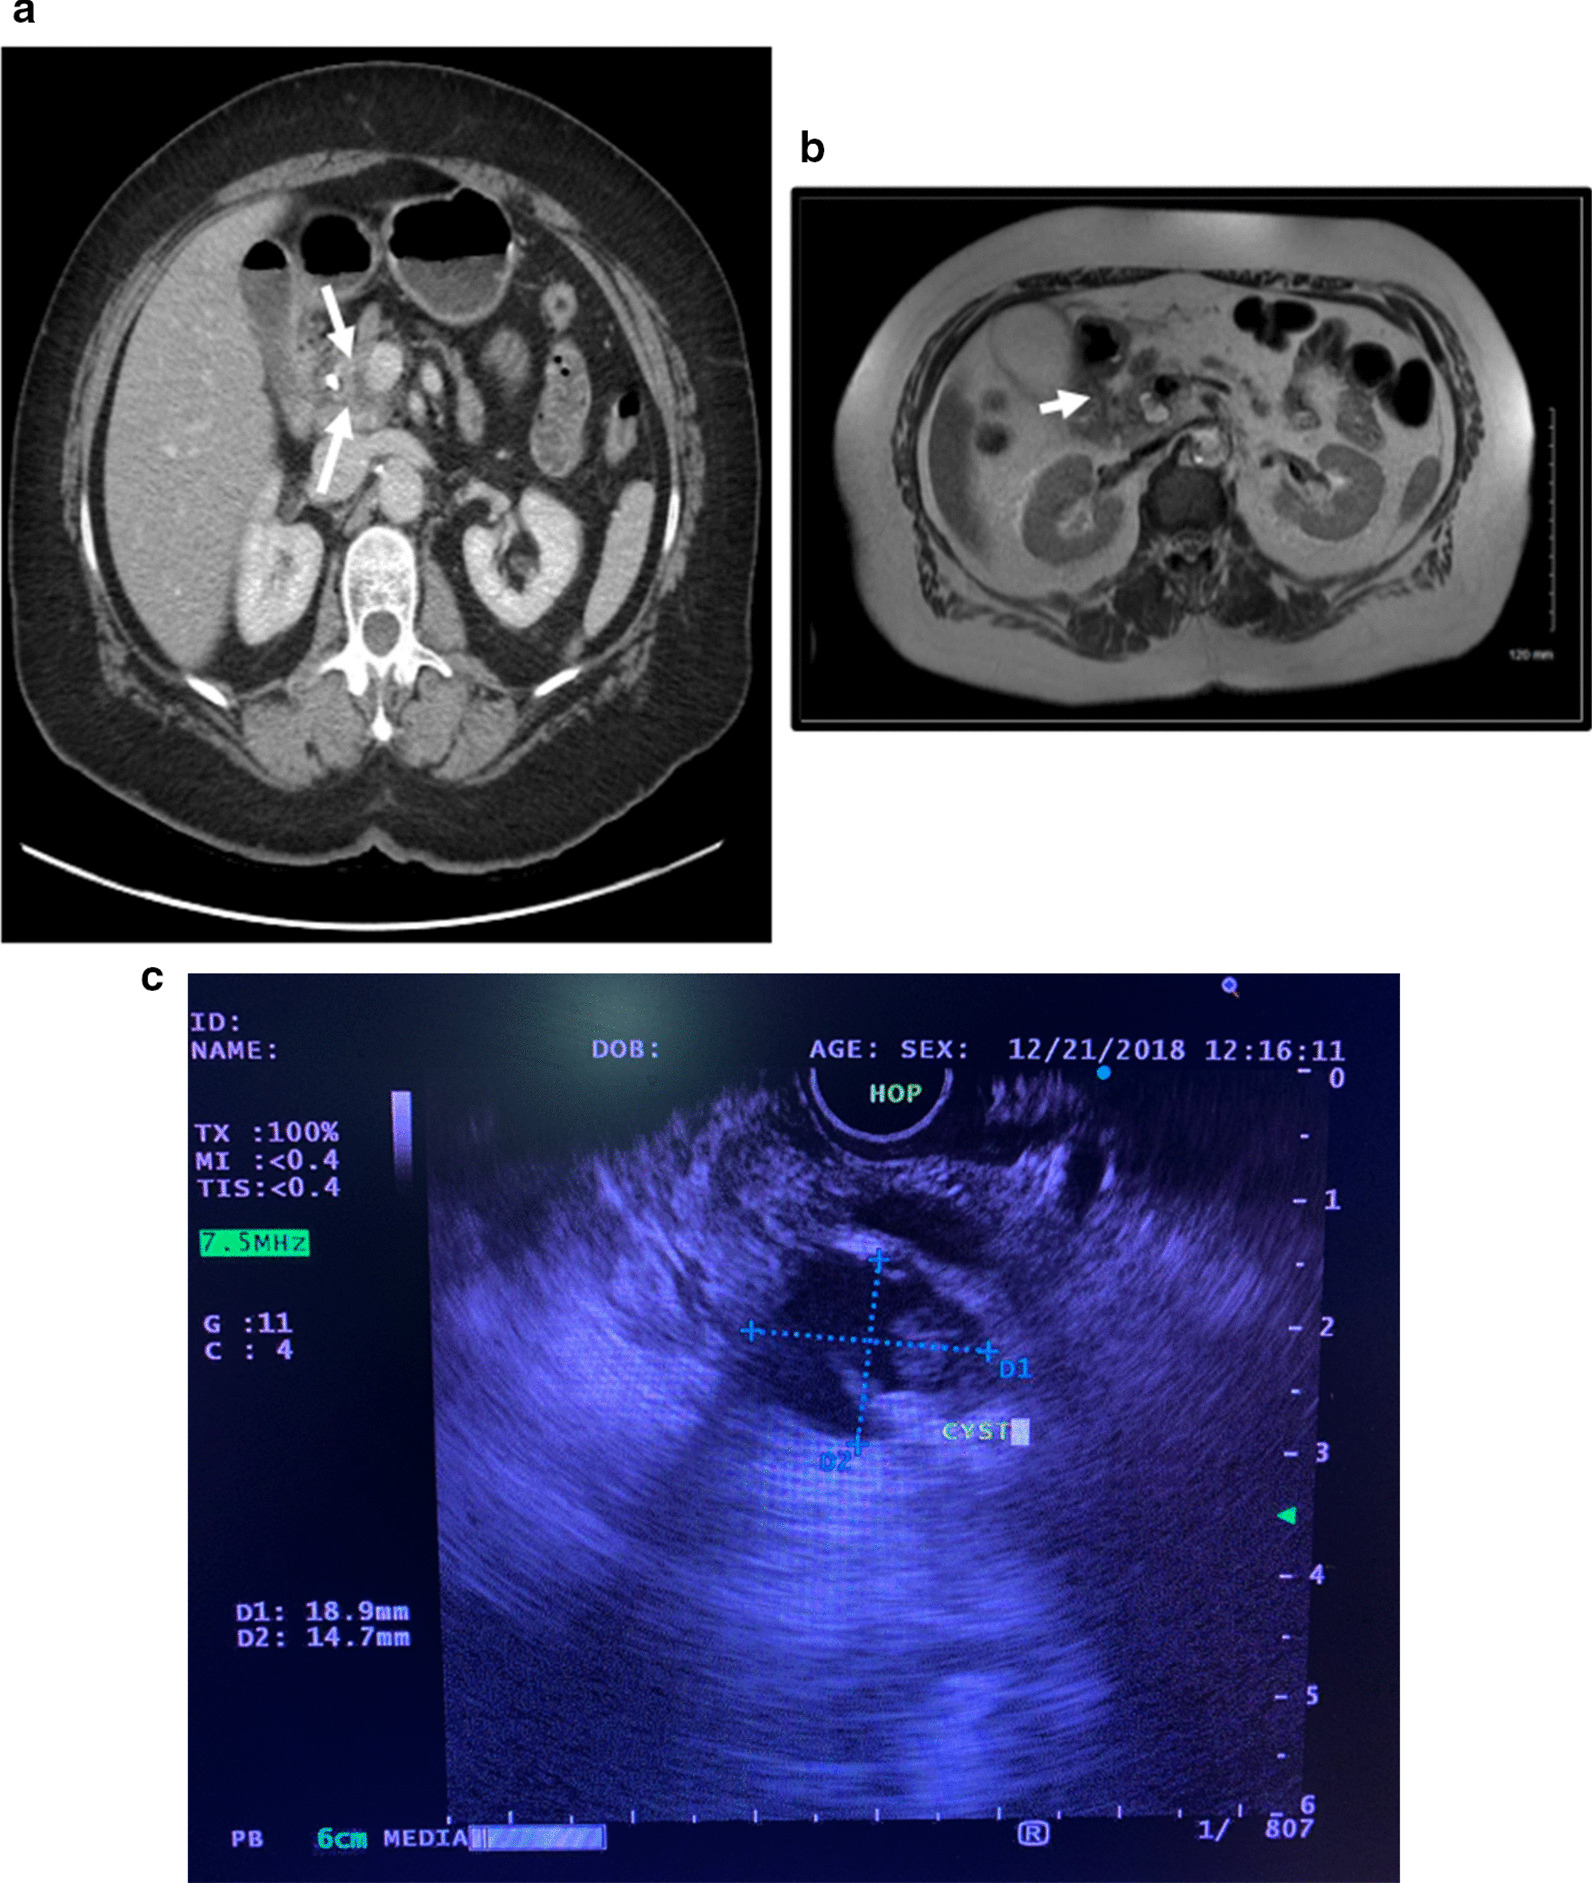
a CT scan demonstrating an indeterminate hypodense area in the pancreatic head (arrows) (axial view). b MRI scan demonstrating the lobulated cyst with eccentric mural nodule in the head of the pancreas with effacement of the distal common bile duct. Associated atrophy of the body and tail of the pancreas (axial view). c EUS showing cyst wall with mural nodule (arrow)
